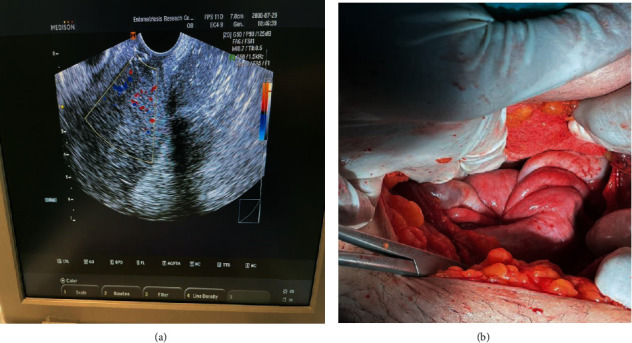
Ultrasound findings indicate the absence of a uterus in the pelvic cavity (a). Confirmation of the absence of uterus in the abdominal cavity after abdominal incision (b).
